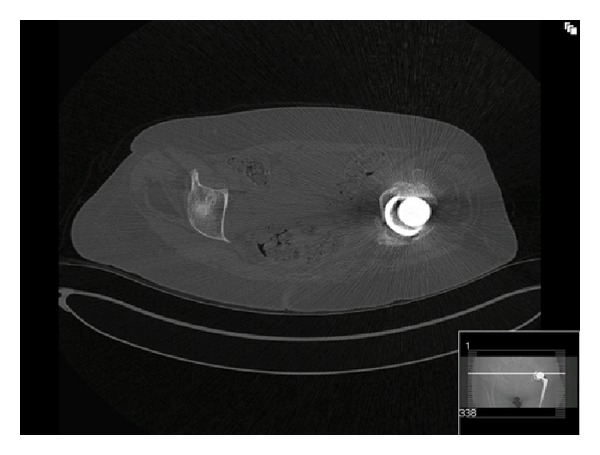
Pelvic CT scan confirming the acetabular fracture and displacement of acetabular component of the total hip replacement.
